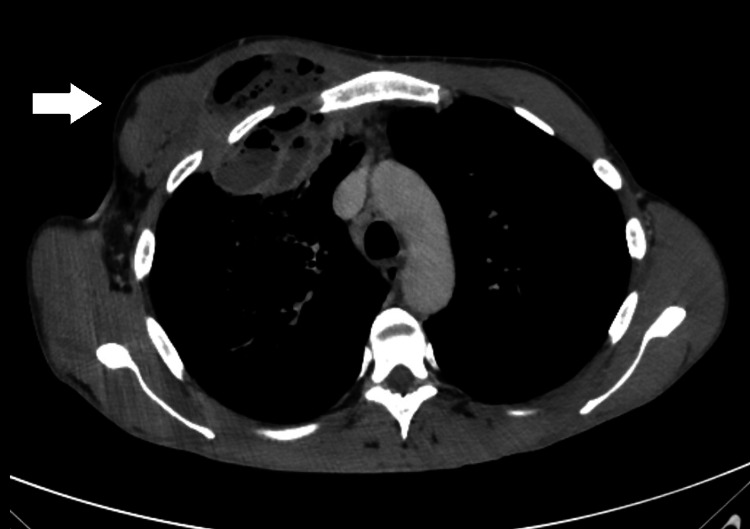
Axial contrast-enhanced chest CT (soft tissue window) demonstrating extension of the infectious process through the intercostal space with formation of an extra-thoracic collection involving the right anterior chest wall, compatible with a pleurocutaneous fistula.
